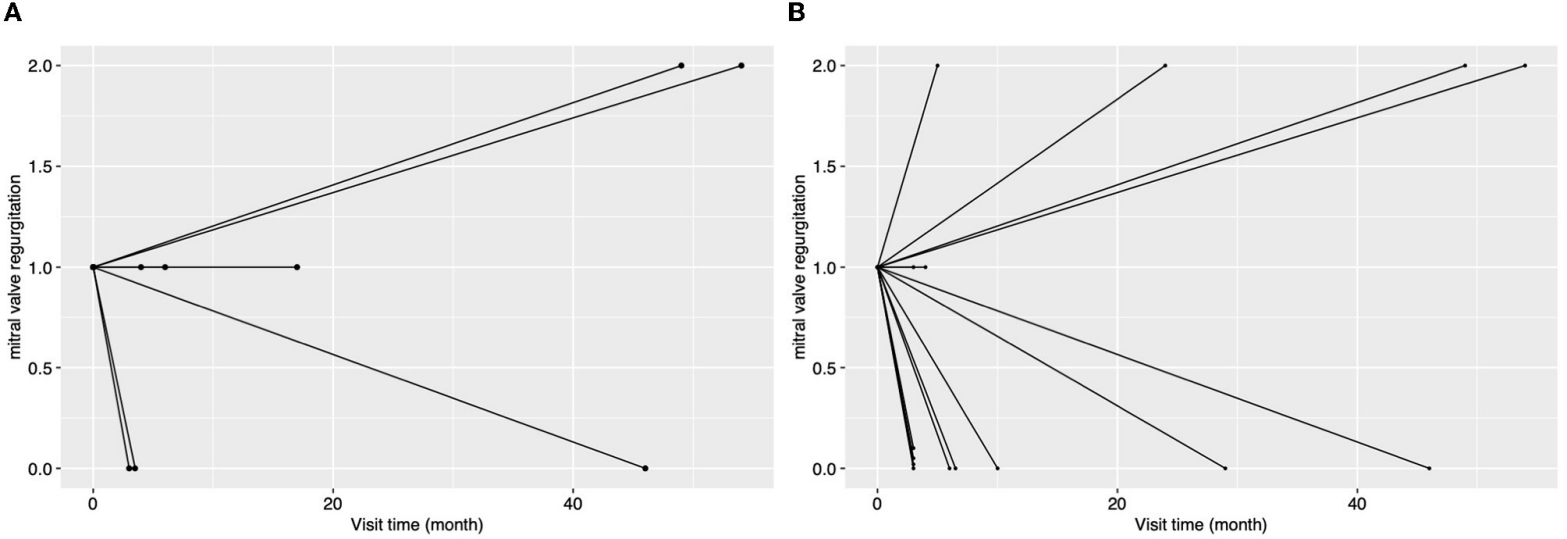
The changes in the degree of MR for patients with rheumatic aortic valve disease [(A), *n* = 8] or with atrial fibrillation [(B), *n* = 15] during the follow-up. In this figure, every line indicates the changes in the degree of mitral regurgitation for a single patient, and the two ends of a line indicate the preoperative echocardiography assessment and the last follow-up echocardiography assessment.
